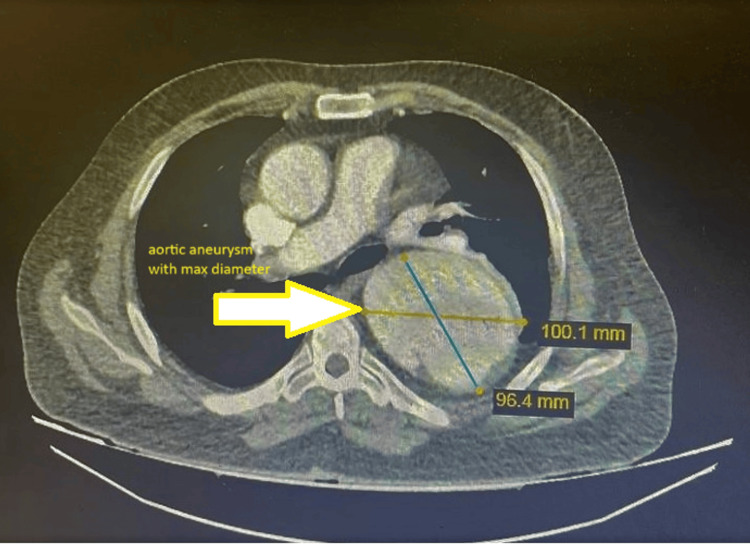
First chest CT scan (pre-operation) This CT shows the thorax, demonstrating a huge thoracic aortic aneurysm (about 100 mm diameter). Such a large dilatation is at very high risk of tear and mandates urgent intervention, typically with TEVAR. CT: computed tomography, TEVAR: thoracic endovascular aortic repair
